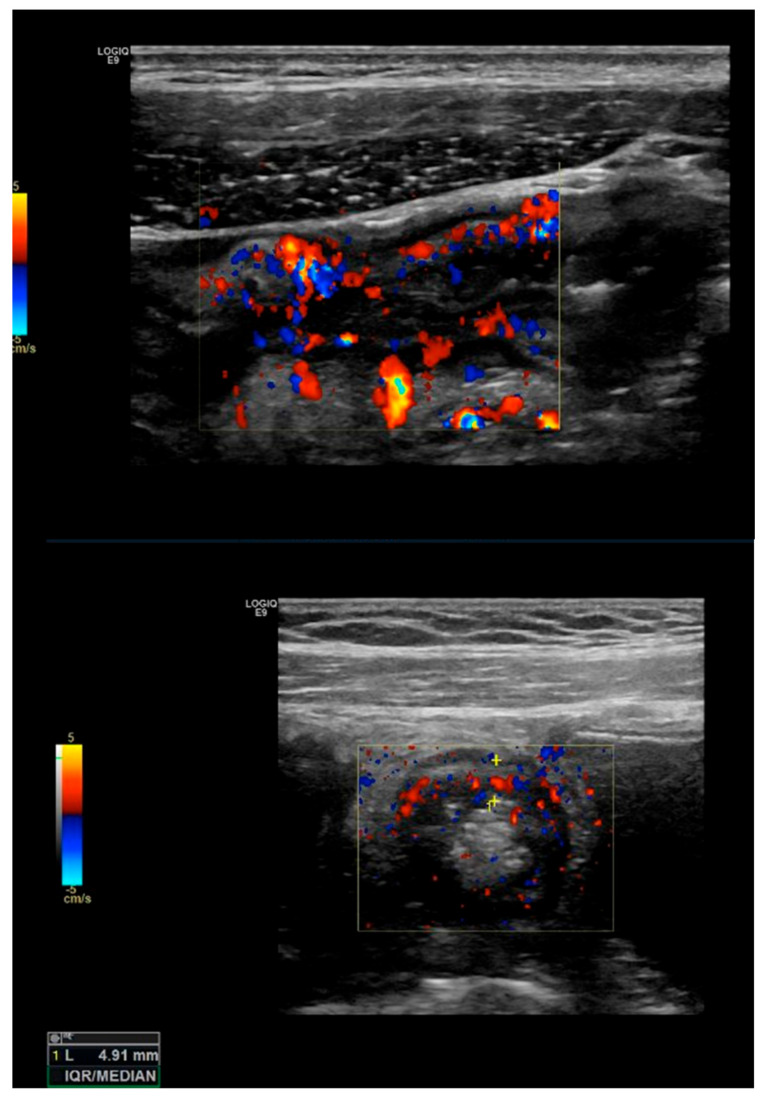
Bowel vascularization: Bowel wall vascularization can be measured by color or power Doppler US. The Limberg score is widely used for the assessment of mural and extramural flow. In the picture, an increased bowel vascularization (Limberg score grade 3) can be visualized in the inflamed bowel.
